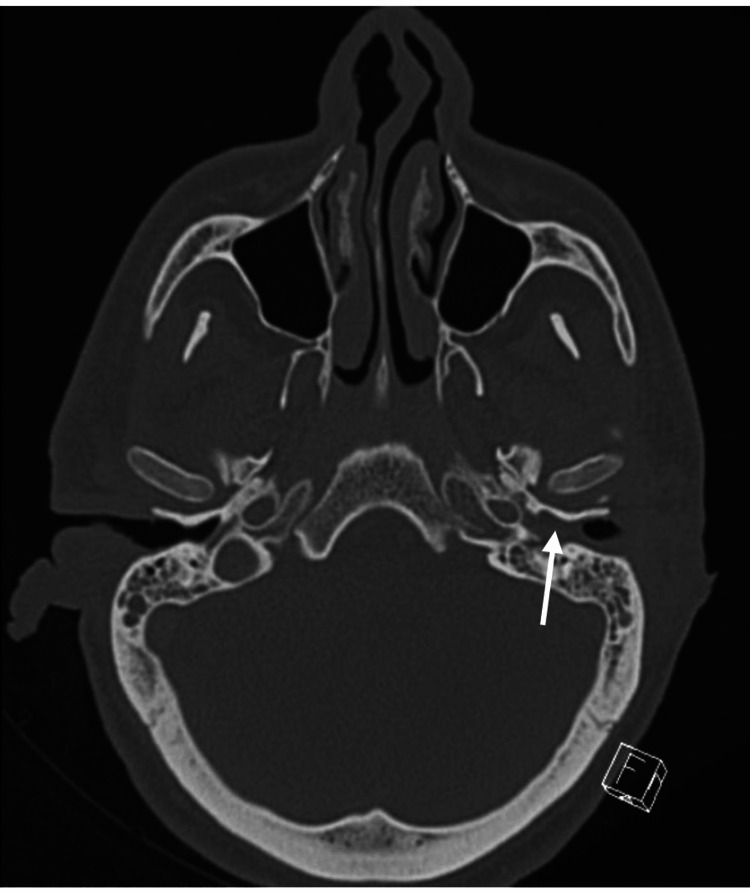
Preoperative CT scan with contrast showing opacity in left canal (white arrow), middle ear cavity, and mastoid cavity without any bone destruction.
